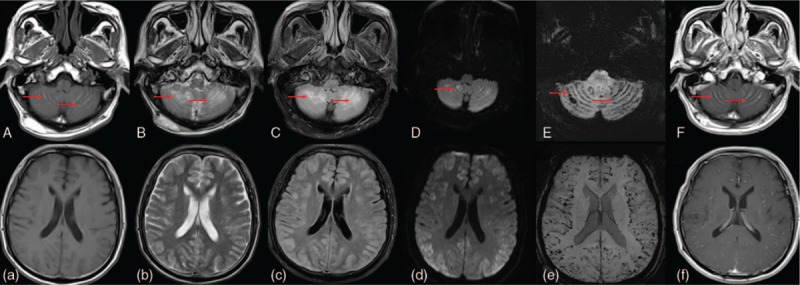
The MRI findings of the patient: (A, a) T1 sequence showed cerebellar sulcus short T1 signal, indicated bleeding (arrowhead). (B, b) T2 showed the long T2 signal of the cerebellum in the focus (arrowhead) and diffuse cerebral cortex swelling; (C, c) flair showed the abnormal signal of the cerebellar cortex (arrowhead) and diffuse cerebral cortex swelling. (D, d) DWI in the cerebellar cortex point flake diffusion limited (arrowhead) and diffuse cerebral cortex swelling, cytotoxic edema was suggested. (E, e) SWI showed diffuse point line like low signal (arrowhead) and diffuse punctate low signal in the cerebral cortex, and extensive haemorrhage was suggested. (F, f) Enhanced MRI showed cerebellar hemispherical linear enhancement (arrowhead) and diffuse leptomeningeal enhancement, and meningeal involvement was indicated.
